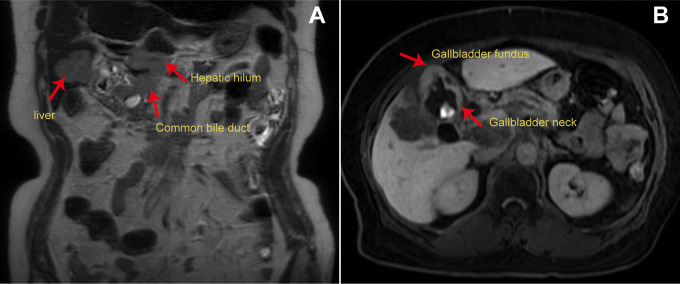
Upper abdominal MRI showing gallbladder carcinoma with metastases to the liver, hepatic hilum, and multiple other sites; (A) Coronal view, (B) Axial view.
